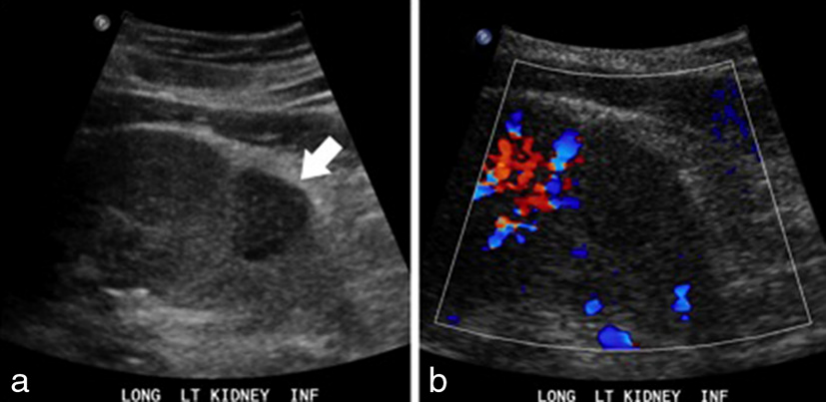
A B-mode longitudinal ultrasound image showing a homogeneous hypoechoic mass (arrow) bulging the renal contour (a) with no appreciable internal flow on Doppler (b).
